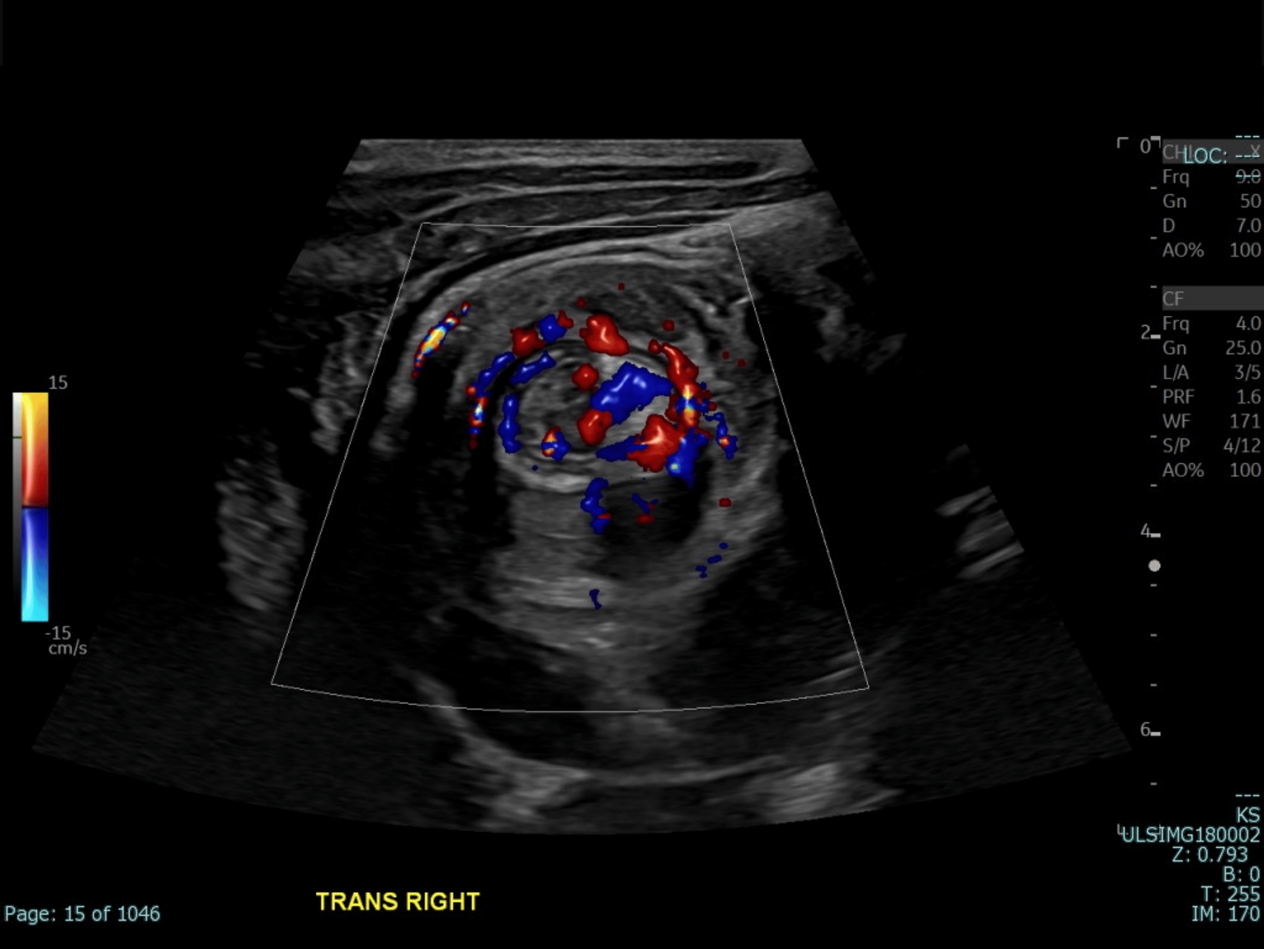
Color Doppler imaging reveals continuous blood flow within the ileocolic segment affected by the intussusception.
